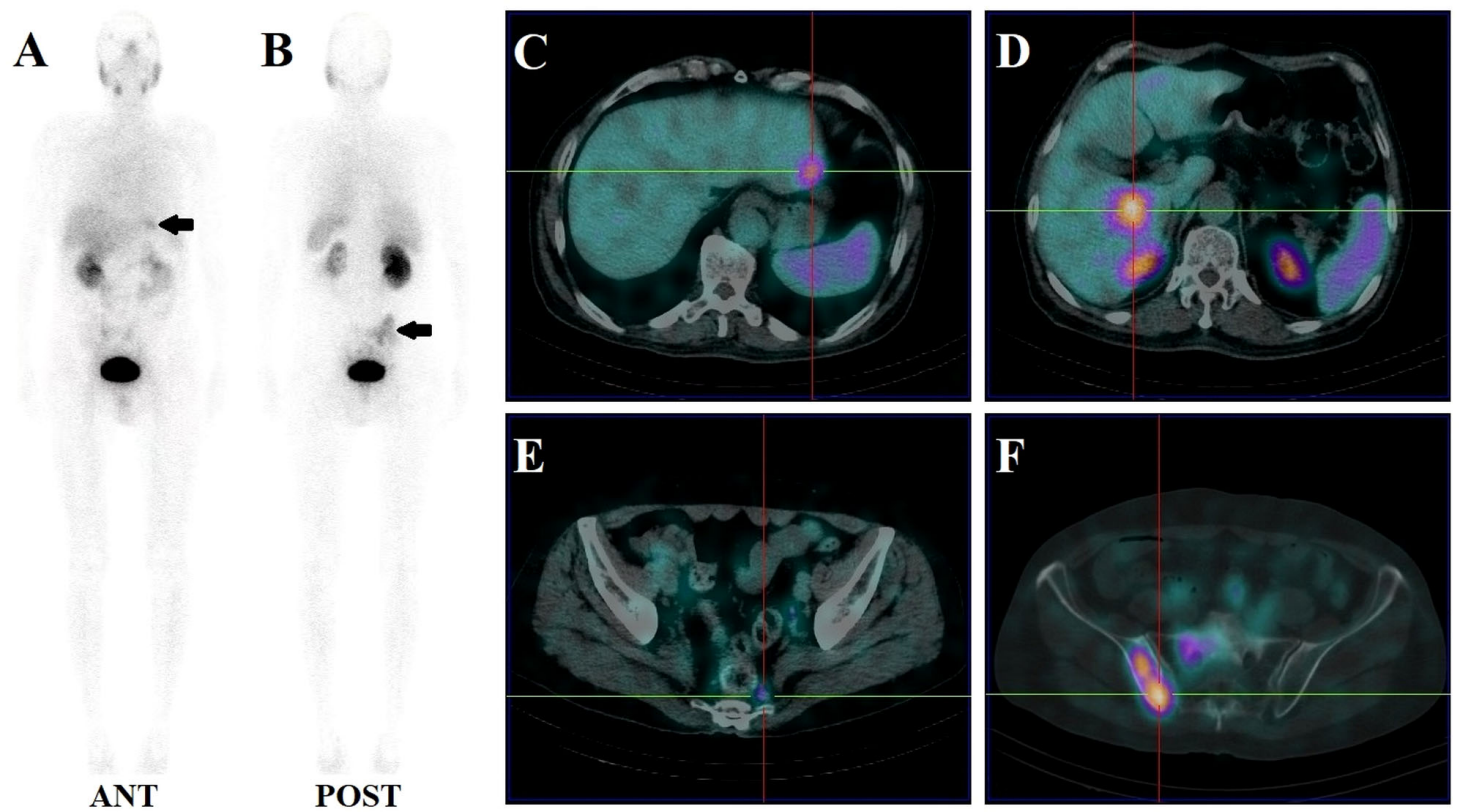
Images of biochemically recurrent prostate cancer in a 70-year-old male with increasing PSA levels after radical prostatectomy (PSA, 187.40 ng/ml; Gleason score, 4 + 5). Whole-body planar scintigraphy (A, B; pointed by black arrows) and fused SPECT/CT images show multiple metastases in the liver (C, D; the highest SUVmax, 4.61), pelvic lymph node (E; SUVmax, 1.84), and sacrum and right iliac bone (F; SUVmax, 6.82). The patient received chemotherapy and his PSA level decreased to 23.73 ng/ml.
